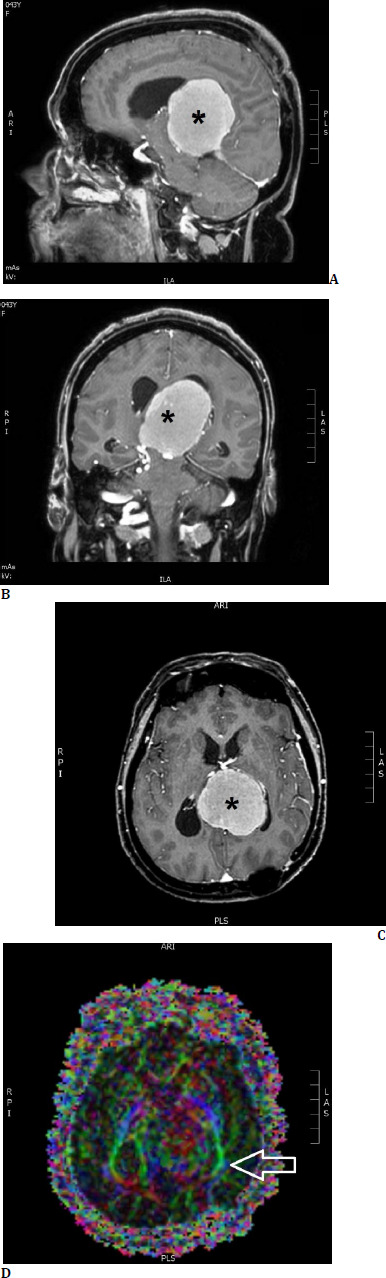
Brain MRI of our first patient, T1-weighted sequence with intravenous contrast, showing a large almost centrally located falcotentorial meningioma (*). A. sagittal section; B. coronal section; C. transverse section; D. tractography showing distortion of the fibers of the left optic radiation (arrow)
